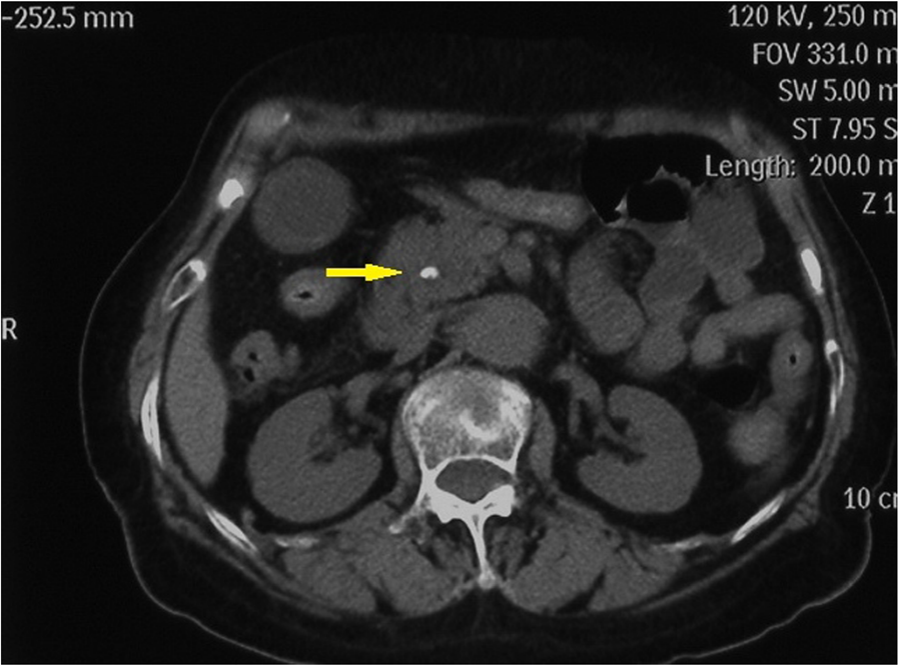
An abdominal computerized tomography (CT) scan demonstrating dilatation of the extrahepatic bile duct with a stone at the lower CBD
